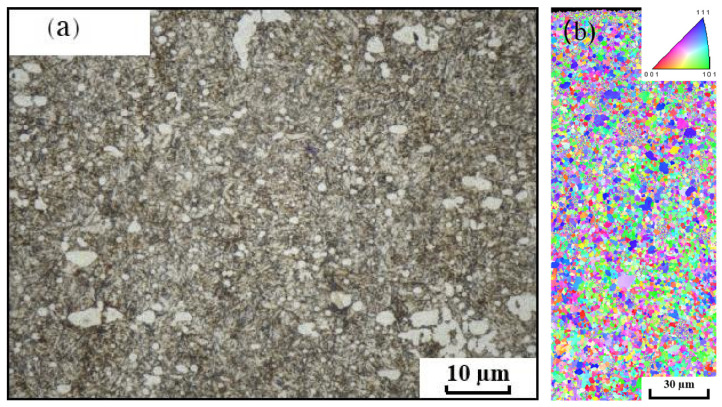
Microstructure of Cr12Mo1V1 die steel components after six ultrasonic impacts: (a) USR-3 equivalent component metallographic structure, (b) USR-3 equivalent component EBSD inverse pole figure.
